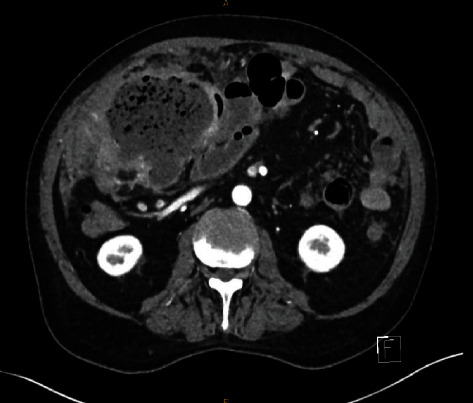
Pre-operative axial CT angiogram revealing relationship of the faecaloma to the ileocolic vessels.
